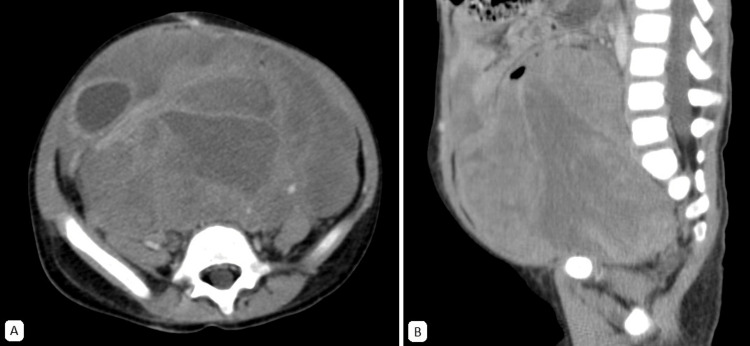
Abdominal CT scan, venous-phase. An extensive, predominantly solid, lobulated mass with heterogeneous contrast enhancement is observed. Hypodense areas, likely indicative of a cystic and/or necrotic component, measure approximately 10 x 14 x 12 cm. The epicenter is located in the pelvic cavity, extending into the abdominal cavity and exerting mass effect on peritoneal structures. A. Axial plane B. Sagittal plane
